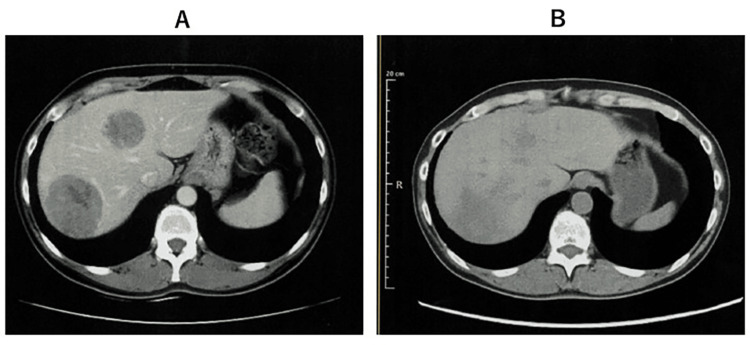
Change of liver metastases over time (A) Abdominal CT after one month of irradiation showing marked shrinkage of liver metastases. (B) Abdominal CT after the fifth dose of WT1-DC showing the disappearance of liver metastases.
